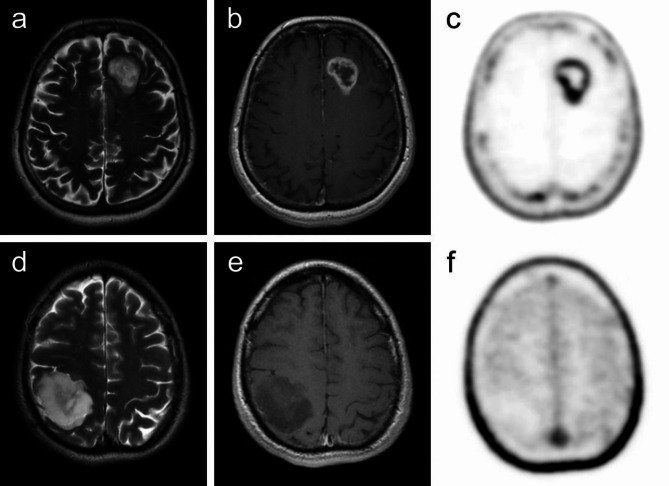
True positive PET/MRI with [^18^F]FLT in a patient with glioblastoma grade IV, IDH wildtype (a-c) and a true negative finding in a patient with low-grade glioma (diffuse astrocytoma grade II, IDH mutant) (d-f). A high grade glioma located in the left frontal lobe is heterogeneously hyperintense on T2-weighted image (a), similar to a low-grade glioma in the right parietal lobe (d). As expected, the high-grade tumour demonstrates strong, mostly peripheral enhancement on contrast-enhanced T1-weighted image (b) and high uptake of [^18^F]FLT on PET (c); conversely, no enhancement (e) or [^18^F]FLT uptake (f) can be seen in a patient with a low-grade glioma
